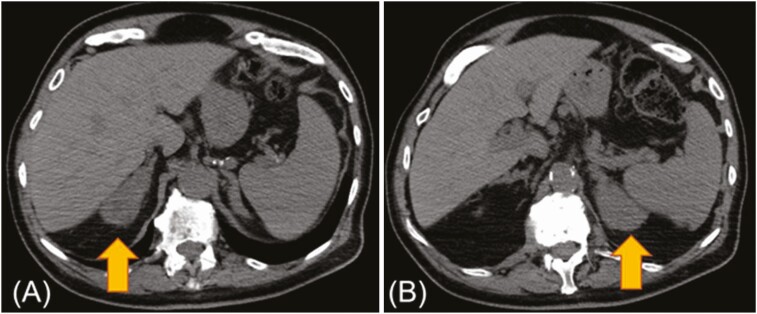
Computed tomography identified enlarged (A) right adrenal gland and (B) left adrenal gland on the axial images (arrows) in patient 2 with nonclassic congenital adrenal hyperplasia.
